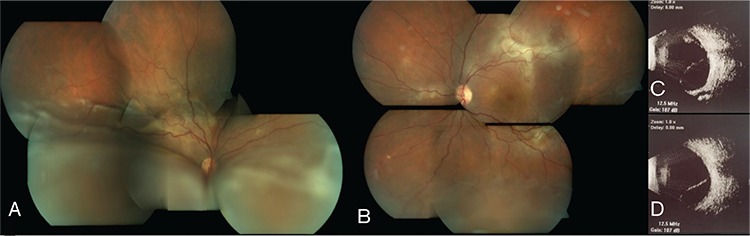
Fundus photography and ultrasonography (USG) images from the patient’s right and left eyes. A) Bullous retinal detachment extending to the superotemporal vascular arcade in the right eye; B) Serous retinal detachment in the inferior periphery and subretinal fibrin visible at the inferior and superior temporal arcades and in the nasal quadrant in the left eye; C) Retinal detachment in the inferior and superior quadrants on the USG in the right eye; D) Retinal detachment in the inferior quadrant on USG in the left eye
